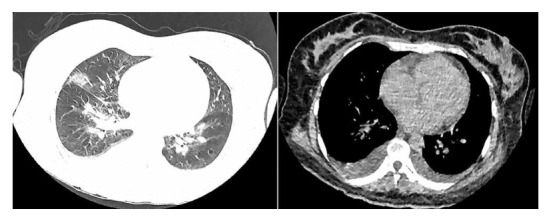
Chest CT scan without contrast with bilateral pleural effusion that resolved in the convalescent phase, no lesions in lung parenchyma.
